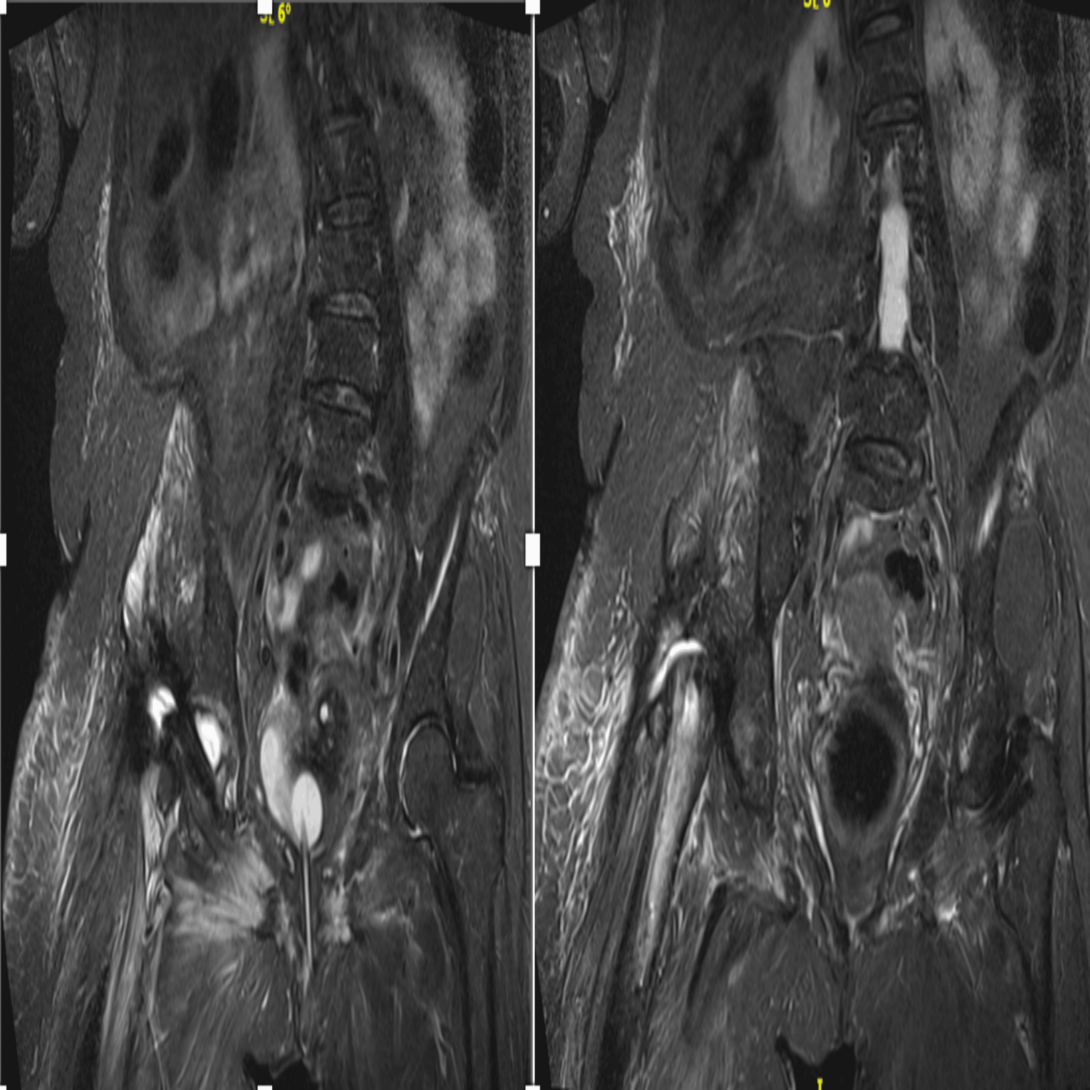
MRI prior to definitive reimplantation MRI: magnetic resonance imaging
